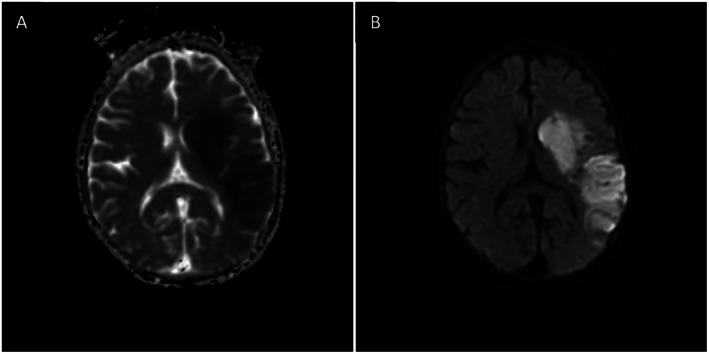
Diffusion‐weighted MRI (A, B). (A) DWI showing marked diffusion restriction in the left corpus striatum and insular cortex (arrowheads). (B) Corresponding ADC map demonstrating low signal in the same regions, confirming true diffusion restriction and acute infarction.
